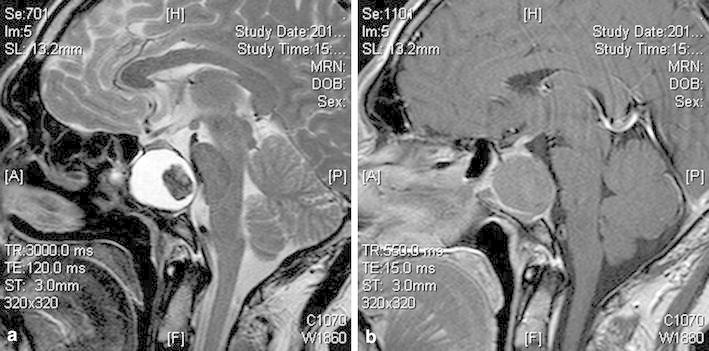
a On T2 weighted image, the central part of the mass was hypo-intense, the peripheral part was hyper-intense; b contrast enhanced T1-weighted MRI showed the mass was of slight rim enhancement. The sella turcica was enlarged and the sphenoidal sinus was greatly diminished
